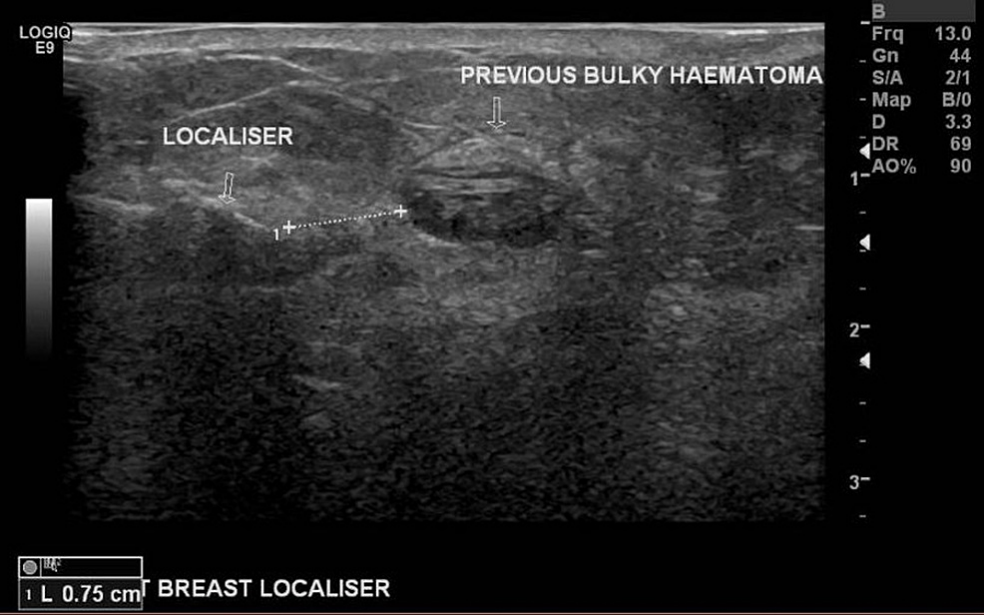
Preoperative right breast ultrasound scan showing the cancerous lesion after localization using a radiofrequency identification (RFID) tag
